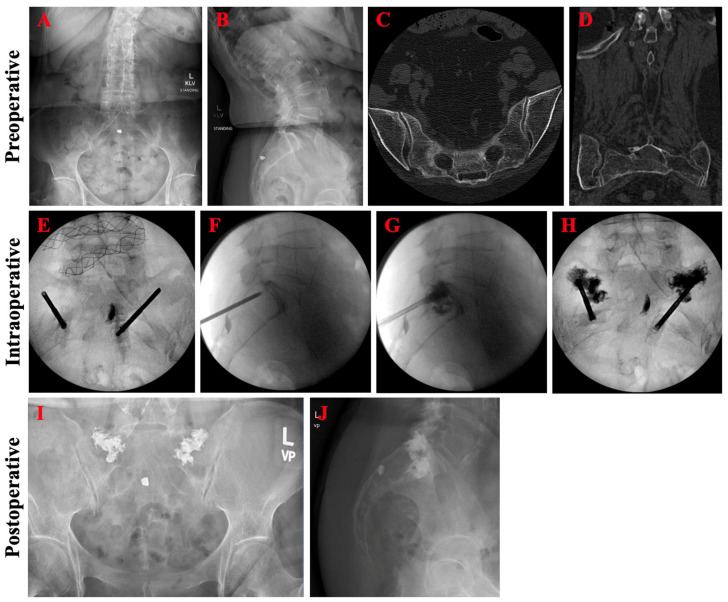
Pre-operative, intraoperative, and post-operative imaging for patient case 1. Pre-operative anteroposterior (A) and lateral (B) lumbosacral spine radiographs demonstrated degenerative spondylolitic changes of the lumbar vertebrae. Axial (C) and coronal (D) CT confirmed bilateral sacral fractures centered around S3. Intraoperative (E–H) imaging during bilateral short-view sacroplasty revealed adequate cement deposition at the fracture site. Post-operative anteroposterior (I) and lateral (J) lumbosacral spine radiographs showed good distribution of the cement without extravasation.
